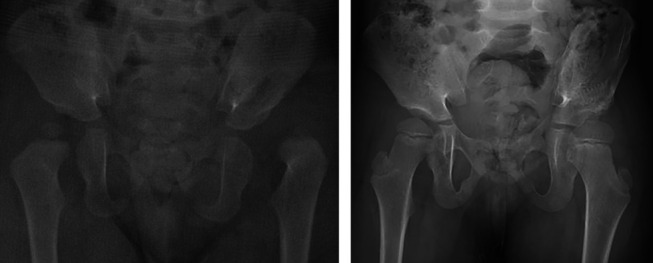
Postoperative development of the capital femoral epiphysis in the left hip in a female DDH patient who underwent OR and DA without PFO at an operative age of 19 months. (c): Preoperative AP pelvic radiograph showing a smaller capital femoral epiphysis on the left side; (d): 39-month postoperative AP pelvic radiograph showing a larger maximum transverse diameter of the capital femoral epiphysis on the left side (*r* = 1.42).
